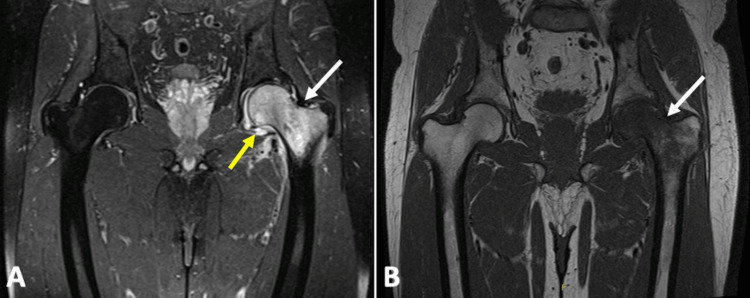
MRI scan performed after three months of symptoms On T2-weighted images(A), hyperintense signal is noted in the proximal femur (white arrow), along with evident joint effusion (yellow arrow). Hypointense signal is observed on T1-weighted images (B) in the same location, with preservation of the joint space.
